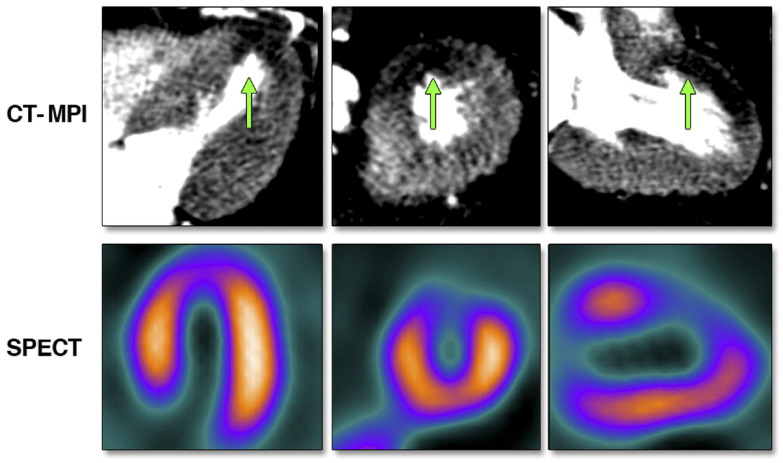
Stress perfusion defect, indicated by the green arrows, in the mid- and distal anterior wall during adenosine stress computed tomography-myocardial perfusion imaging (top) and the perfusion defect in the same wall segments using single photon emission tomography-myocardial perfusion imaging (bottom). Reprinted from JACC: Cardiovascular Imaging, Volume 3, Issue 10, Tamarappoo, B.K. et al. [177], Comparison of the Extent and Severity of Myocardial Perfusion Defects Measured by CT Coronary Angiography and SPECT Myocardial Perfusion Imaging, pages 1010–1019, Copyright 2010, with permission from Elsevier.
